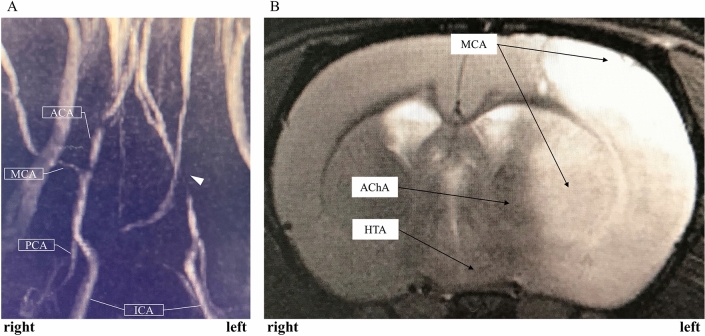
MRI 24 h after the creation of the zirconia MCA occlusion model. The zirconia ball did not affect the MRI. (A) MR angiography detected the impaired blood flow in the left MCA. (B) T2-weighted MRI showed infarction only in the MCA perfusion area, but not in the AchA and HTA. *AChA* anterior choroidal artery, *HTA* hypothalamic artery, *MCA* middle cerebral artery, *MRI* magnetic resonance imaging.
